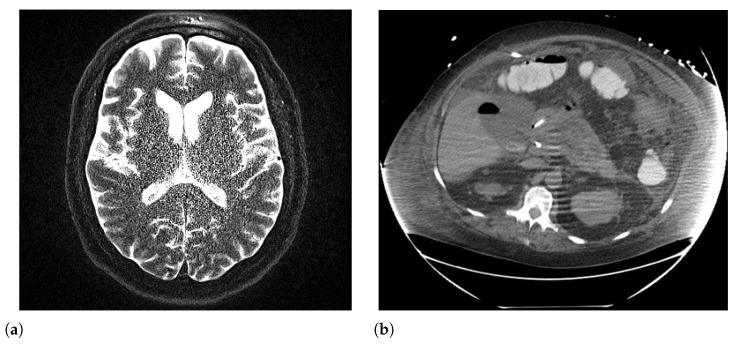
(a) MRI of the human brain corrupted by noise [63]; (b) streak artifacts generated by large body habitus [64].
